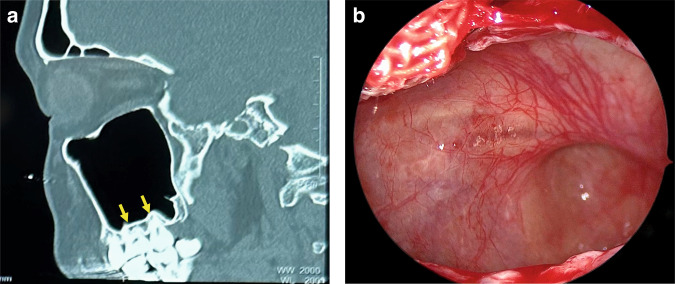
The relationship between the maxillary sinus floor and upper teeth. a CT image shows the roots of maxillary molars are located closest to the maxillary sinus floor. b Endoscopic image shows the maxillary sinus floor protrudes toward the oral cavity
